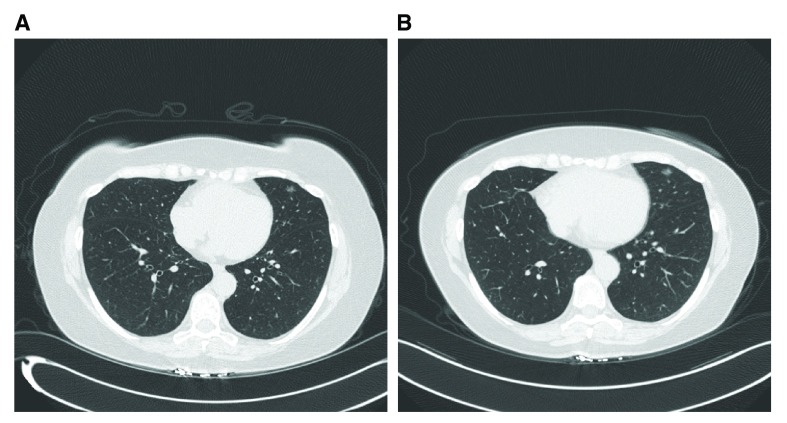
A 67-year-old woman, former smoker with a 9 mm ground glass opacity (GGO) in the lingula. The nodule has changed minimally over 3 years and is currently being followed with annual computed tomography (CT). If this is a cancer, it is likely to be an adenocarcinoma
